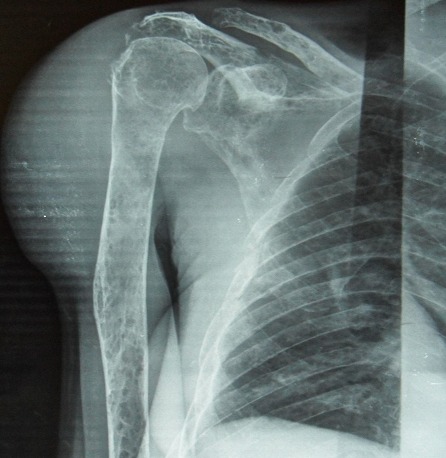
Radiographie de face de l'humérus droit montrant une tuméfaction en regard de l’épiphyse et diaphyse humérale correspondant à un plasmocytome cutané: déminéralisation diffuse avec un amincissement des corticales; lésions ostéolytiques multiples à type de geode
